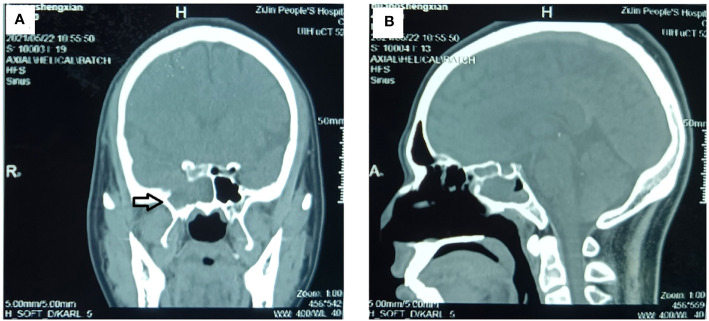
Preoperative CT examination of the paranasal sinus. The CT examination showed an inhomogeneous mass in the right sphenoid sinus cavity, and steolytic bone destruction and defect in the parietal wall of sphenoid sinus. (A) Sagittal image and (B) coronal image. (The mass is marked with a black arrow.).
